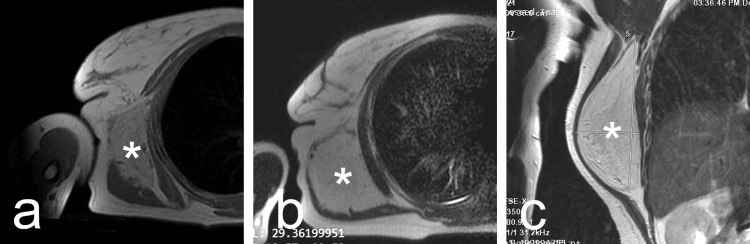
(a-c) Magnetic resonance imaging presented a well-defined, round mass between the serratus anterior and latissimus dorsi muscles with high signal intensity usually slightly less than that of the subcutaneous fat (asterisks).
